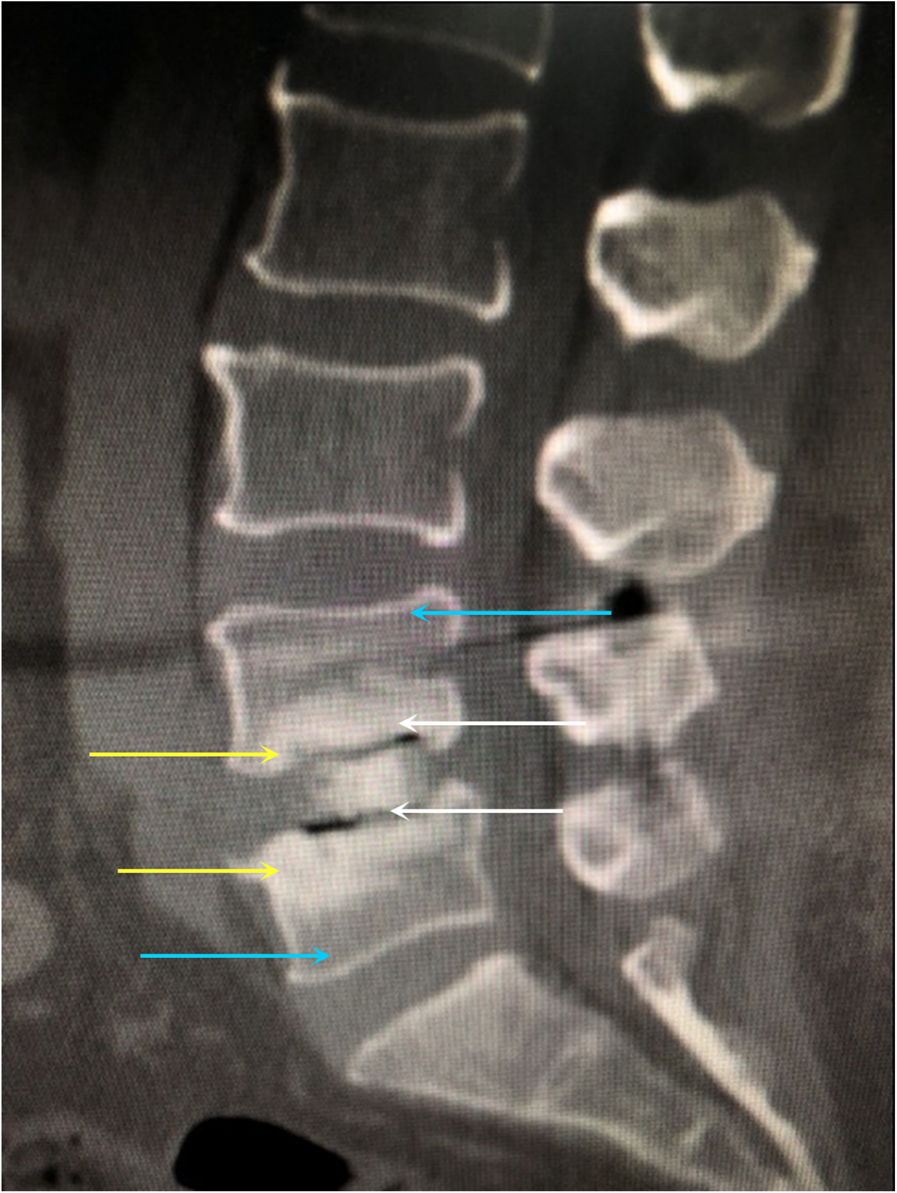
Postoperative spine CT scan. There was potential translucency present at top and bottom of graft(L4/5 cage). There were vertebral compression fractures in both lower endplate and vertebral body of the L4 and upper endplate and vertebral body of the L5. The portion between the two white arrows is the transparent strip. The blue arrow indicates the normal cancellous bone manifestation of the vertebral body. The orange arrow indicates the “hardening”that occurs after a compressive fracture of the cancellous bone inside the vertebral body
